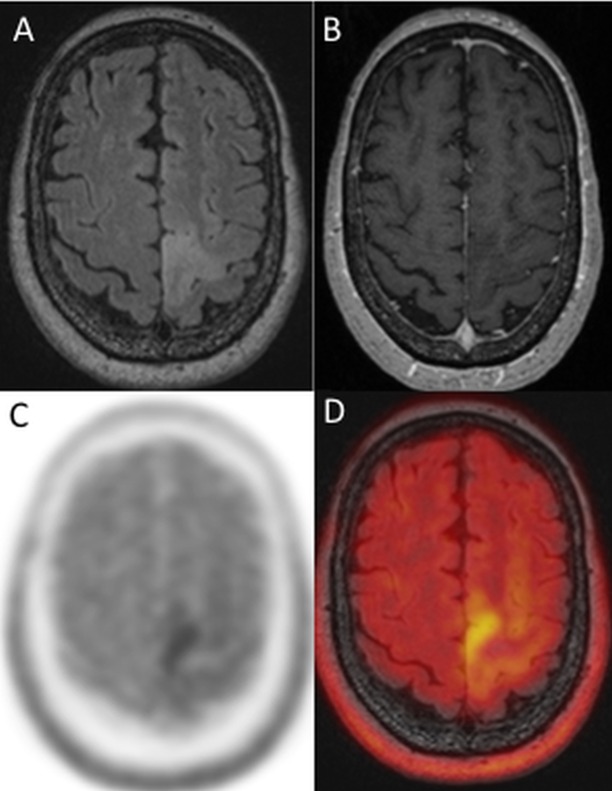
Astrocytoma WHO grade II. 71-year old female patient with left sided pre-/central FLAIR—hyperintensity (A) without contrast enhancement (B). Integrated methionine PET showed a focal pathologic tracer uptake (SUVmax 2.5 T/N, ratio 2.27) of this lesion (C, D (fusion of FLAIR and PET)). Diagnosis was an astrocytoma WHO grade II.
